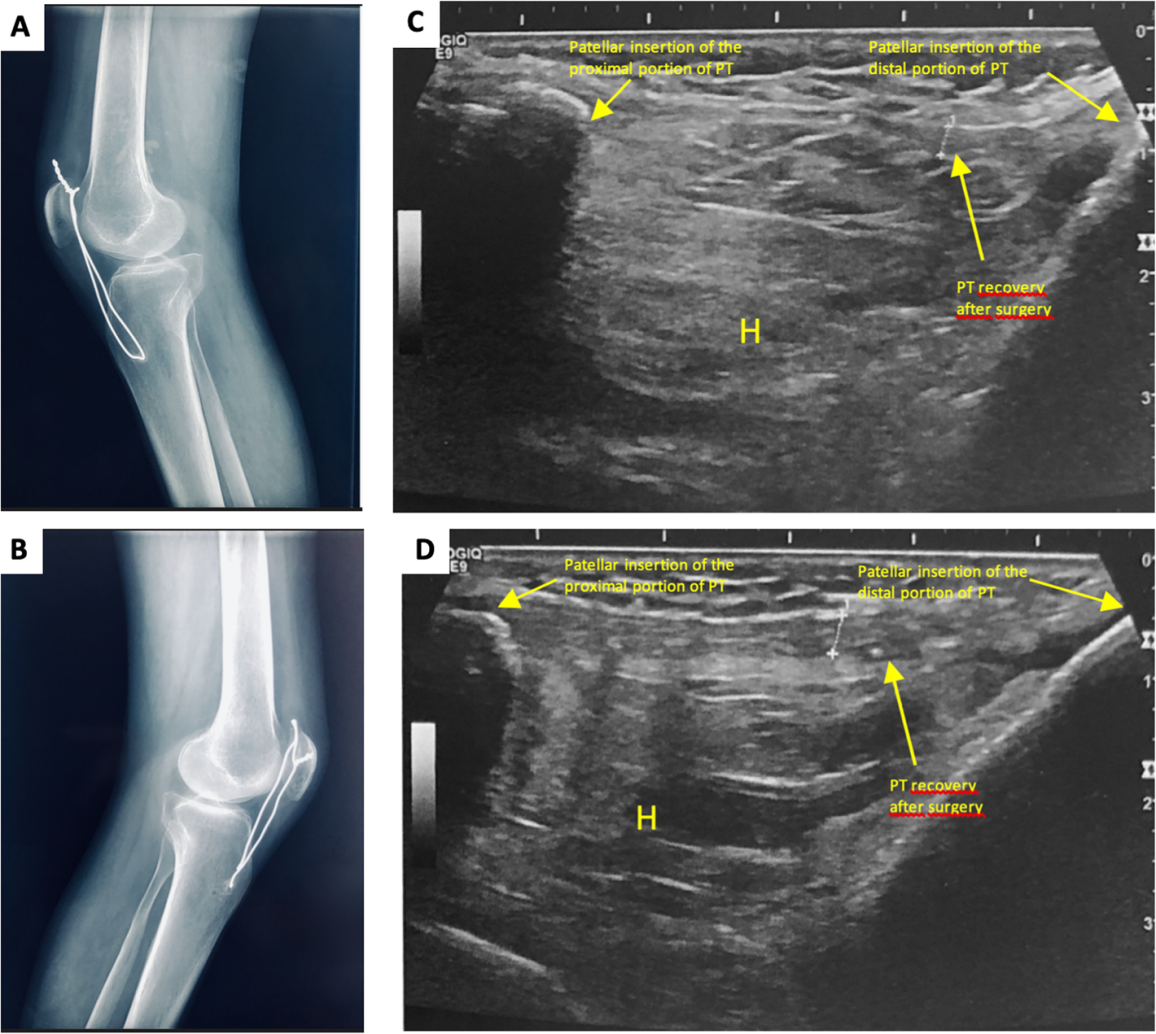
Control x-rays at 3 months showing the recovery of the patellar height with the cerclage wires in the right (a) and left (b) knees. Ultrasound longitudinal scans of the knees at 6 months of follow-up showing recovered patellar tendons in the right (c) and left (d) knees. PT: patellar tendon, H: Hoffa’s fat pad
